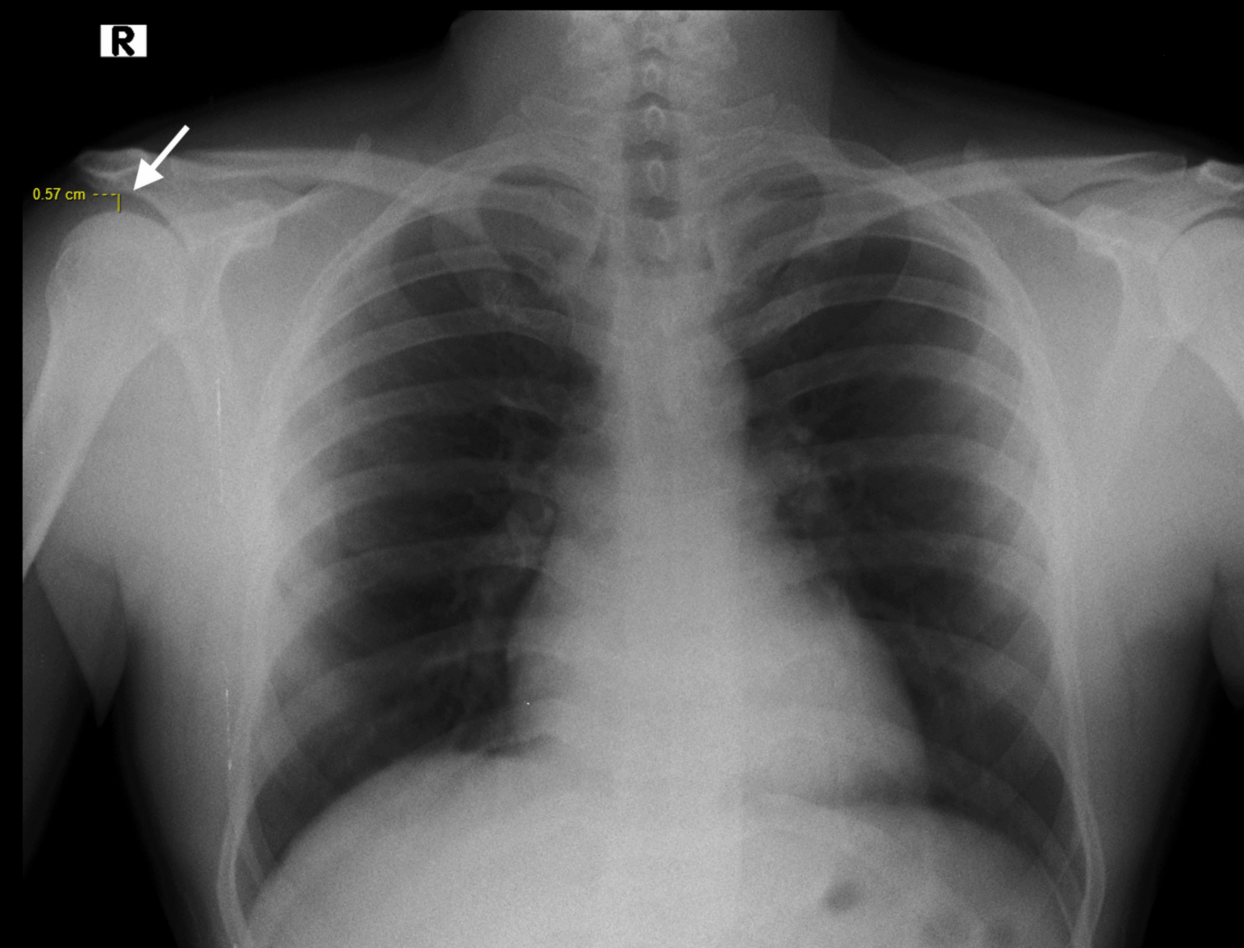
Postero-anterior (PA) view of the chest radiograph shows acromiohumeral index (AHI) - 0.57 mm on the right side.
